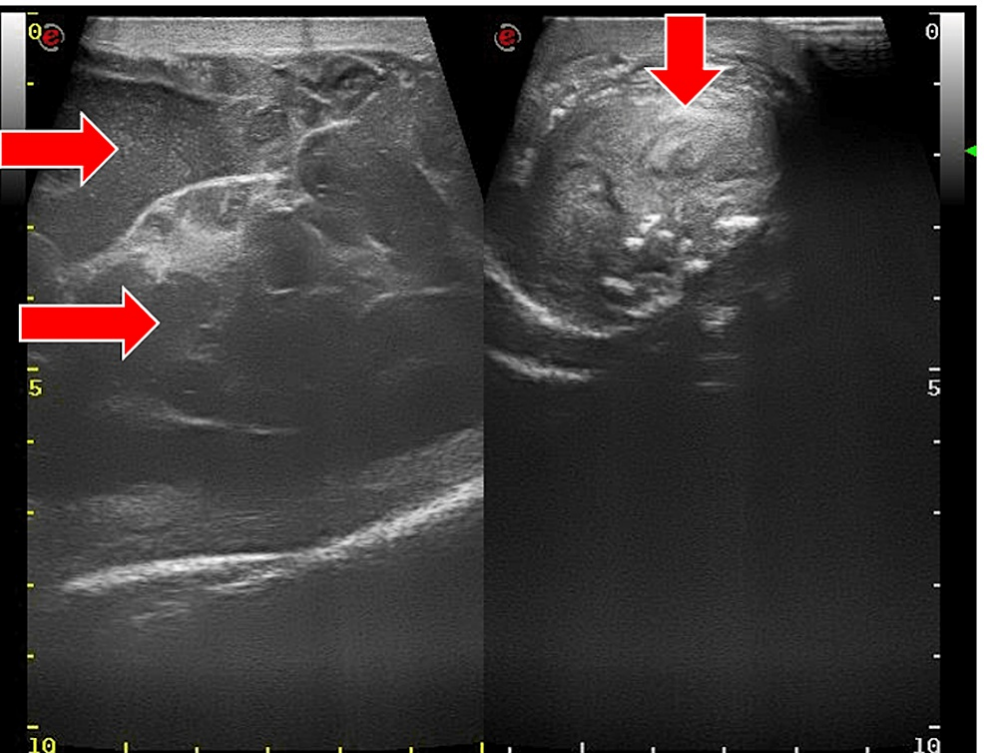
Ultrasound: scrotum - image 1 The image demonstrates heteroechoic mass that is inseparable from the right testis with evidence of a mild amount of echogenic free fluid surrounding the mass lesion
